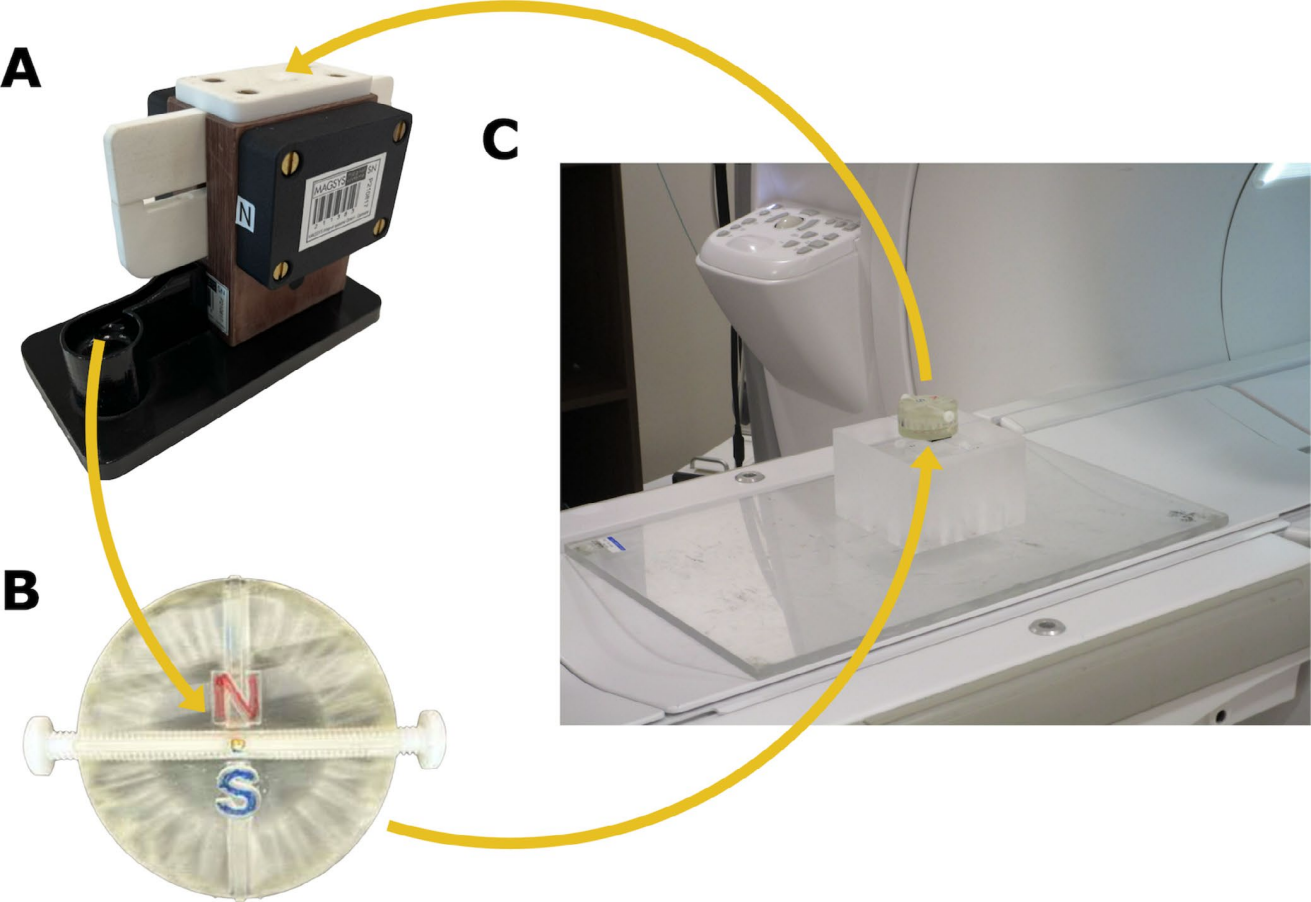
Magnetization strength of permanent magnets pre and post MRI exposure. (A) The magnetization strength (residual flux density) of each permanent magnet was measured using a custom Helmholtz coil measurement device. (B) Each magnet was then affixed at a predetermined angle in a custom fixture. (C) Each magnet was then exposed to MRI‐strength fields and gradients during imaging in the fixture at iso‐center (image shows fixture before movement into iso‐center), after which it was then measured again using the Helmholtz coil measurement device.
